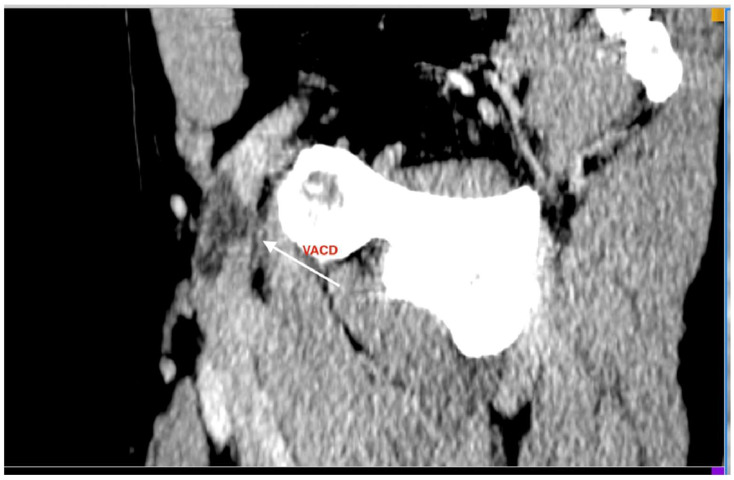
Axial contrast-enhanced computed tomography angiography demonstrating venous adventitial cystic disease; the arrow indicates the cystic lesion.
